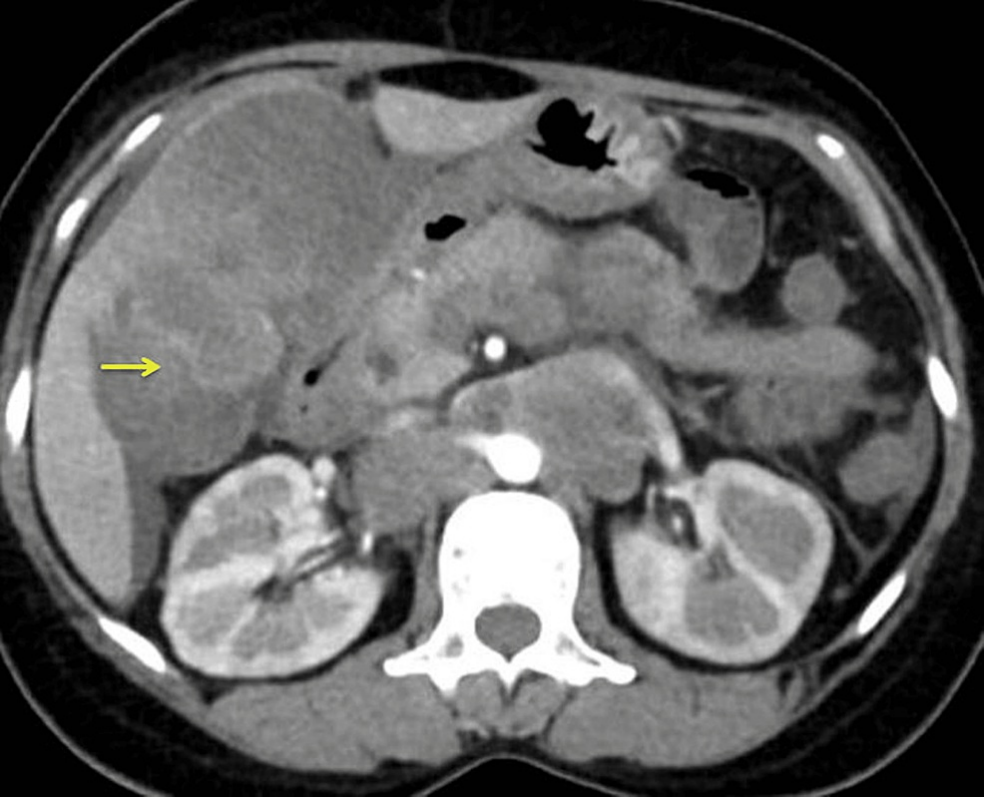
CT of the abdomen shows a large heterogeneous mass in the GB fossa (arrow)
